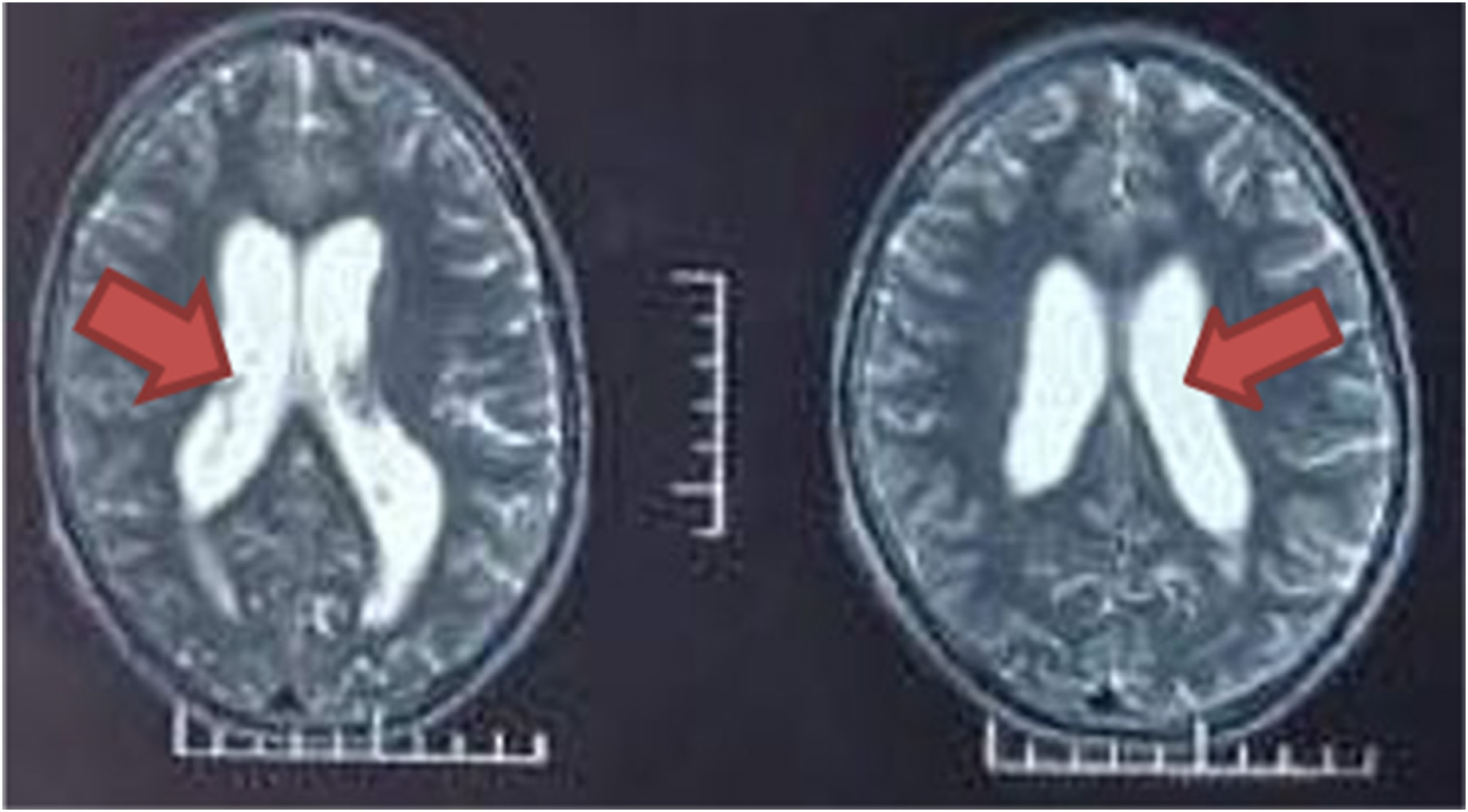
Magnetic resonance imaging (MRI) showing dilated ventricles due to hydrocephalus.
